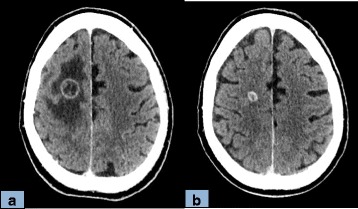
Brain computed tomography scan comparison after the first (a) and the fourth (b) nivolumab infusion. Computed tomography scan, view through the corona radiata 6 days after the first nivolumab injection (a) and after four courses of nivolumab plus oral corticosteroid therapy (b). The large frontal rim-enhanced metastasis decreased substantially in size, from 21 to 9 mm, and the surrounding edema resolved completely
